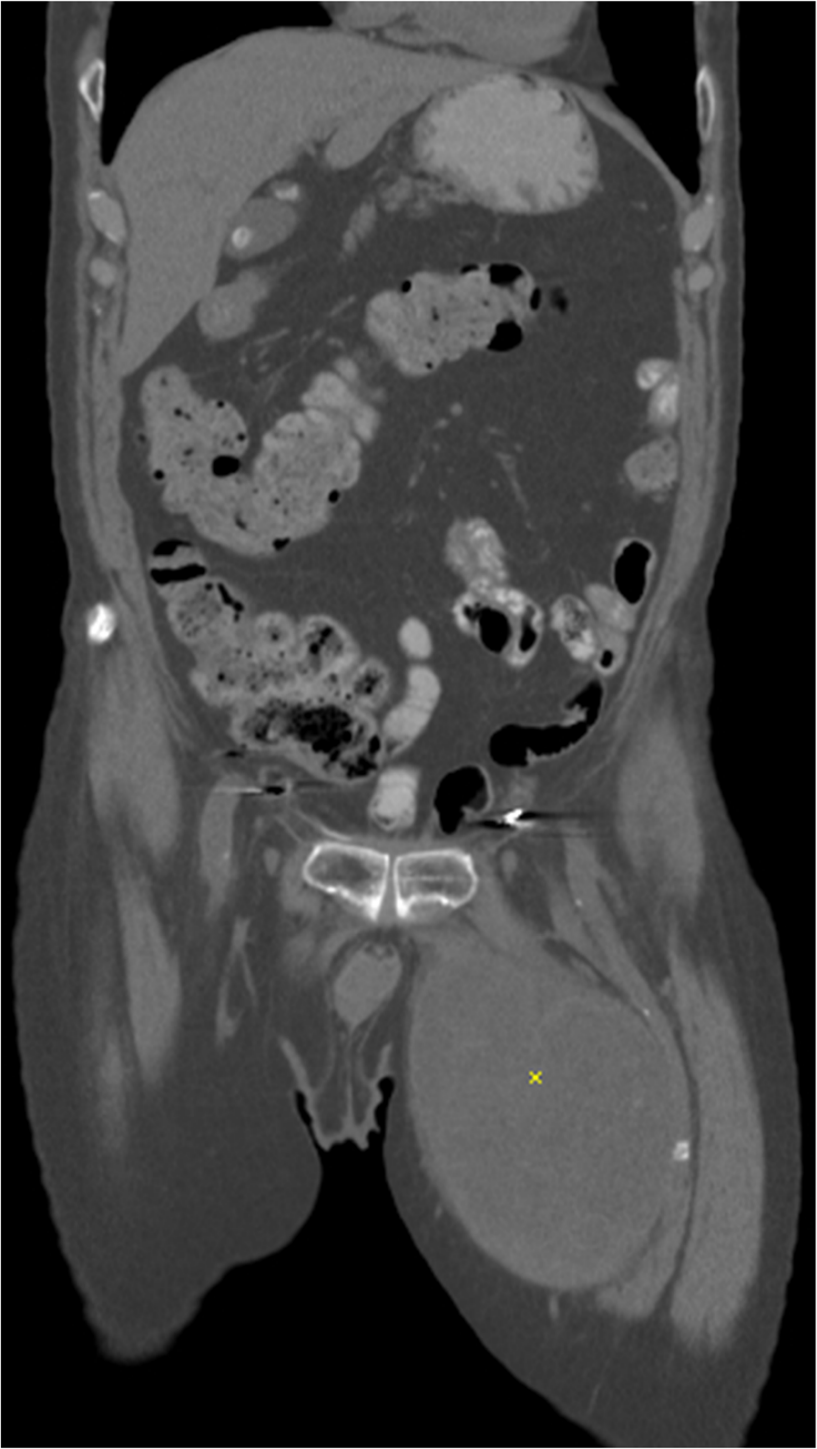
Abdomino-pelvic CT scan showing tumor in the left medial thigh (*).
